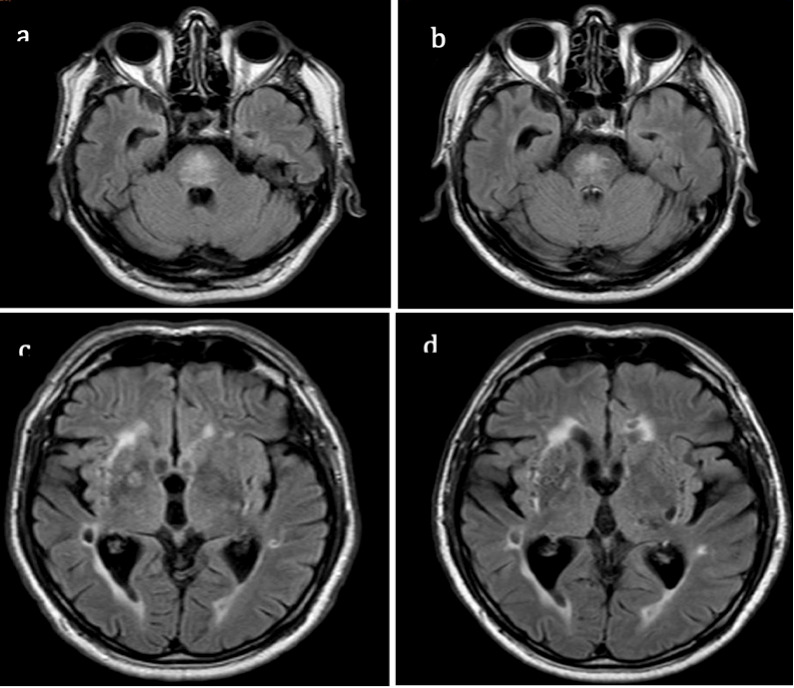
MRI fluid-attenuated inversion recovery (FLAIR) in 2007 (a,c) and in 2014 (b,d). During these years, there was a reduction in the volume of pontine myelinolysis (a,b); the hyperintensities in deep white matter and in deep nuclei are similar (c,d).
